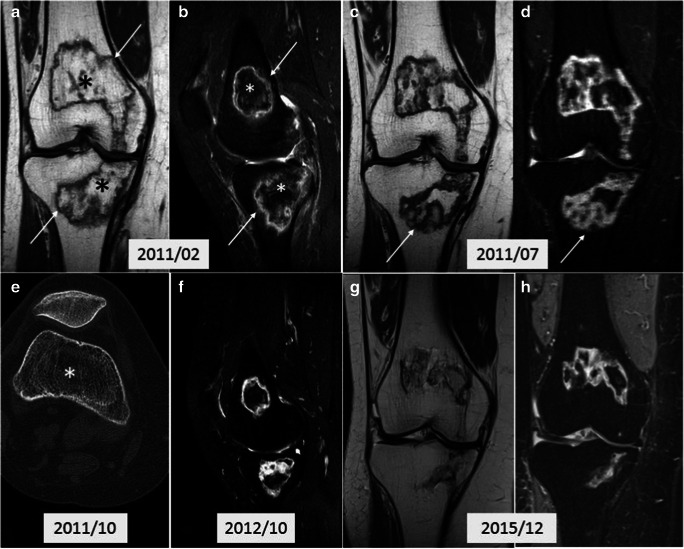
a, b, c, d, e, f, g, h AVN. A 53-year-old woman with pain during mobilization of the right knee; MRI has raised the suspicion of AVN (bone infarct) and we can note the evolution of the findings over 4 years. T1w (a) and proton density fat–suppressed (PD-FS, b) imaging show two areas of altered signal in the distal end of the femur and in the proximal end of the tibia with irregular morphology and inhomogeneous structure (*), with a peripheral rim, hypointense in T1w (*arrows*) and hyperintense in PD-FS (*arrows*) imaging corresponding to the reactive interface along the margin of infarct. Three months after the previous MRI (c, T1w and d, PD-FS), a further increase in the interface (*arrows*), a reduction in the ischemic area and signs of regeneration of the cancellous bone are observed. While the MRI findings are well evident, the CT performed in the same period (e) shows only a limited rarefaction (*) of the cancellous bone. One year later (f, T2w-FS) the infarct outcome area is still evident but smaller compared to previous examinations. Four years later (g, PD and h, STIR) the infarct zone appears less extensive and more shaded, but still present; the interface appears larger. ELMSI had not been detected for all 4 years and the patient progressively underwent an improvement in the clinical situation, with the disappearance of the pain. It is important to underline that ELMSI represents a fundamental indicator of a subchondral fracture and a progression towards epiphyseal collapse, which in this case did not occur
